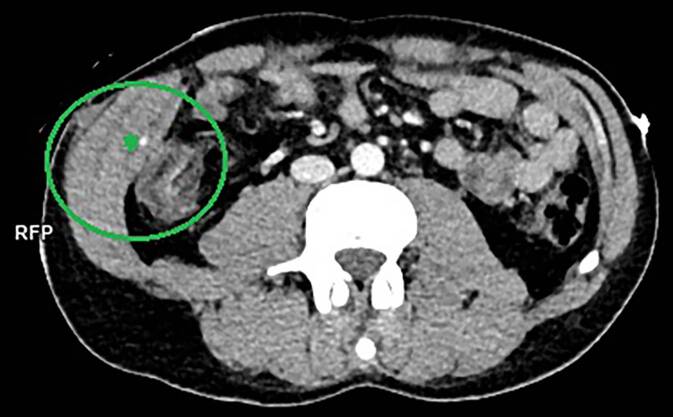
CT-Angiographie (PMCTA). Stichverletzung rechter Unterbauch mit Einblutung in das Weichgewebe, aktiver Muskelblutung (*Stern*) und Eröffnung der Bauchhöhle. Einblutungen in Peritoneum und Darm
